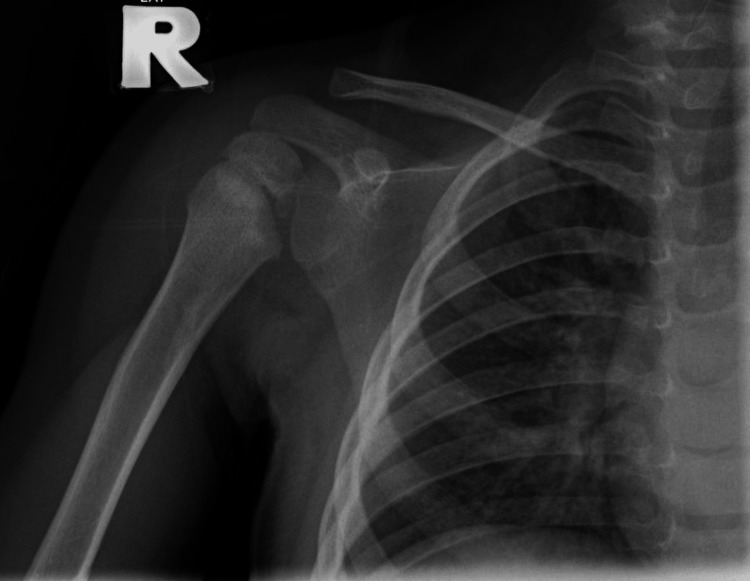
Right shoulder radiograph at one-year follow-up.
